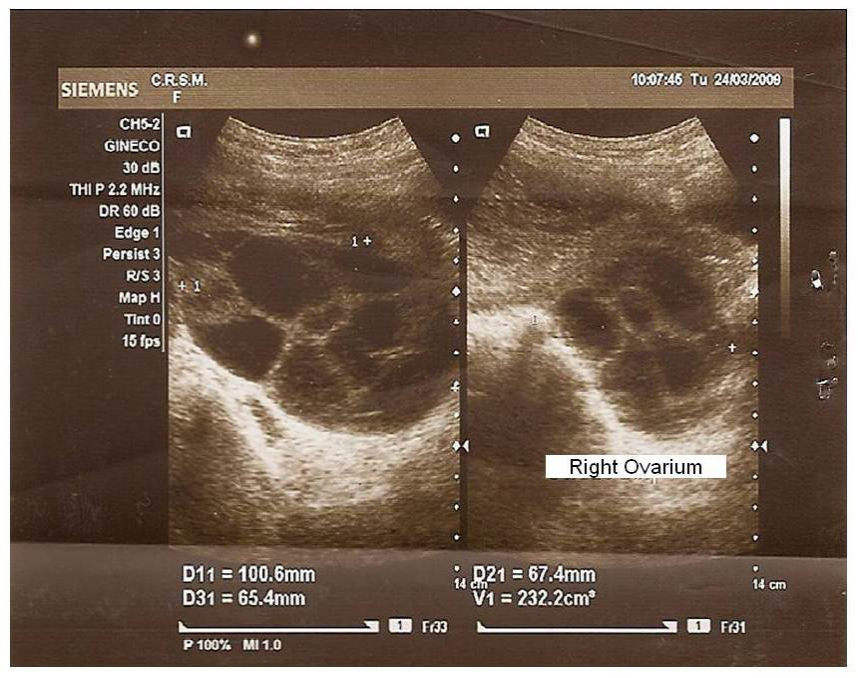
Ultrasound aspect of the right ovary with 232 cc, showing large theca lutein cysts (case 2).
